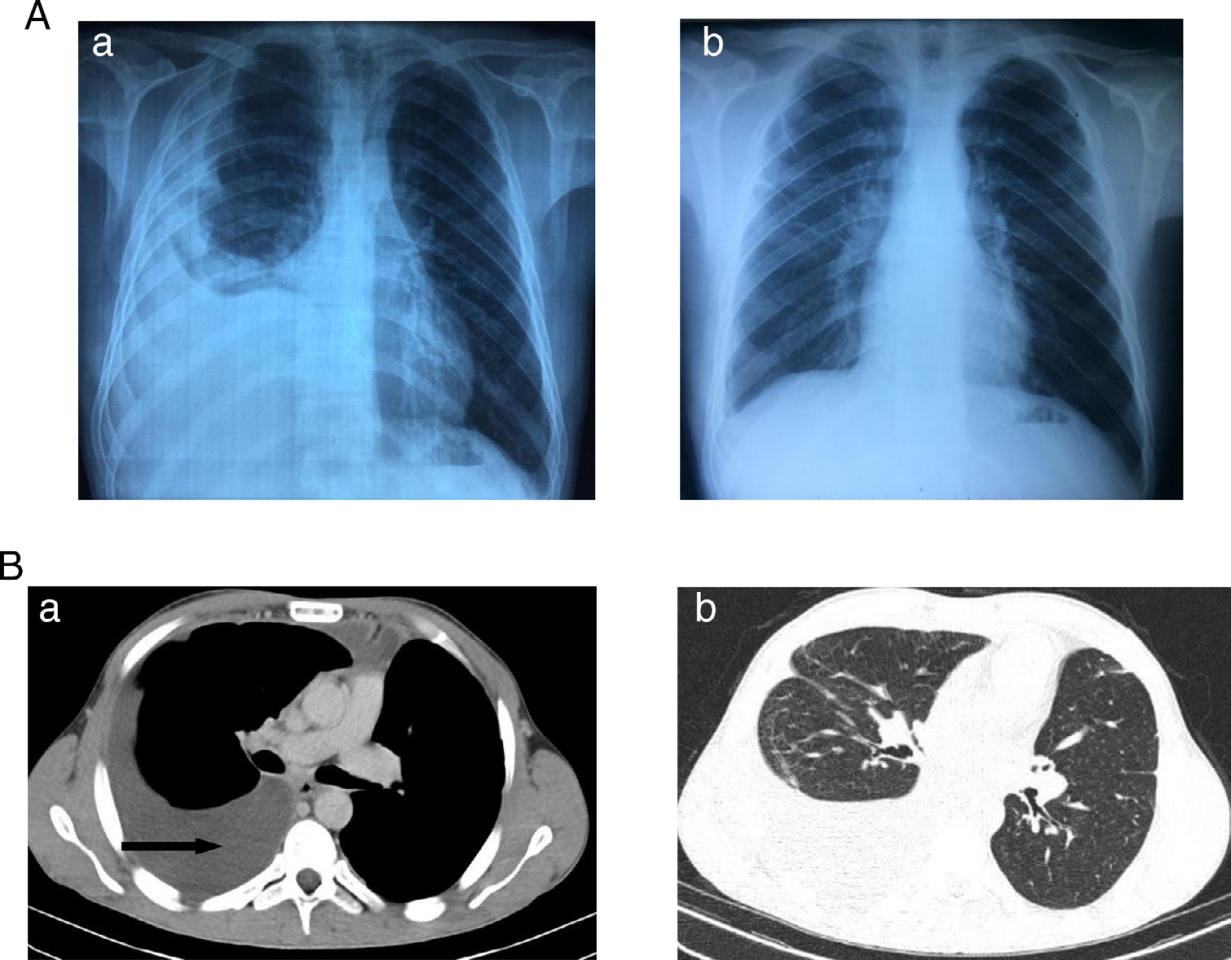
Imaging findings. (A) Frontal chest radiograph. a, Before treatment: an opacity of the lower part of the right hemithorax corresponding to a pleural effusion. b, After video‐assisted thoracoscopic surgery (VATS): no recurrence of pleural effusion. (B) Chest computed tomography (CT) scan: (axial image—a: mediastinal and b: parenchymal windows): a right‐sided pleural effusion (black arrow) without evidence of pleural or parenchymal lesion.
